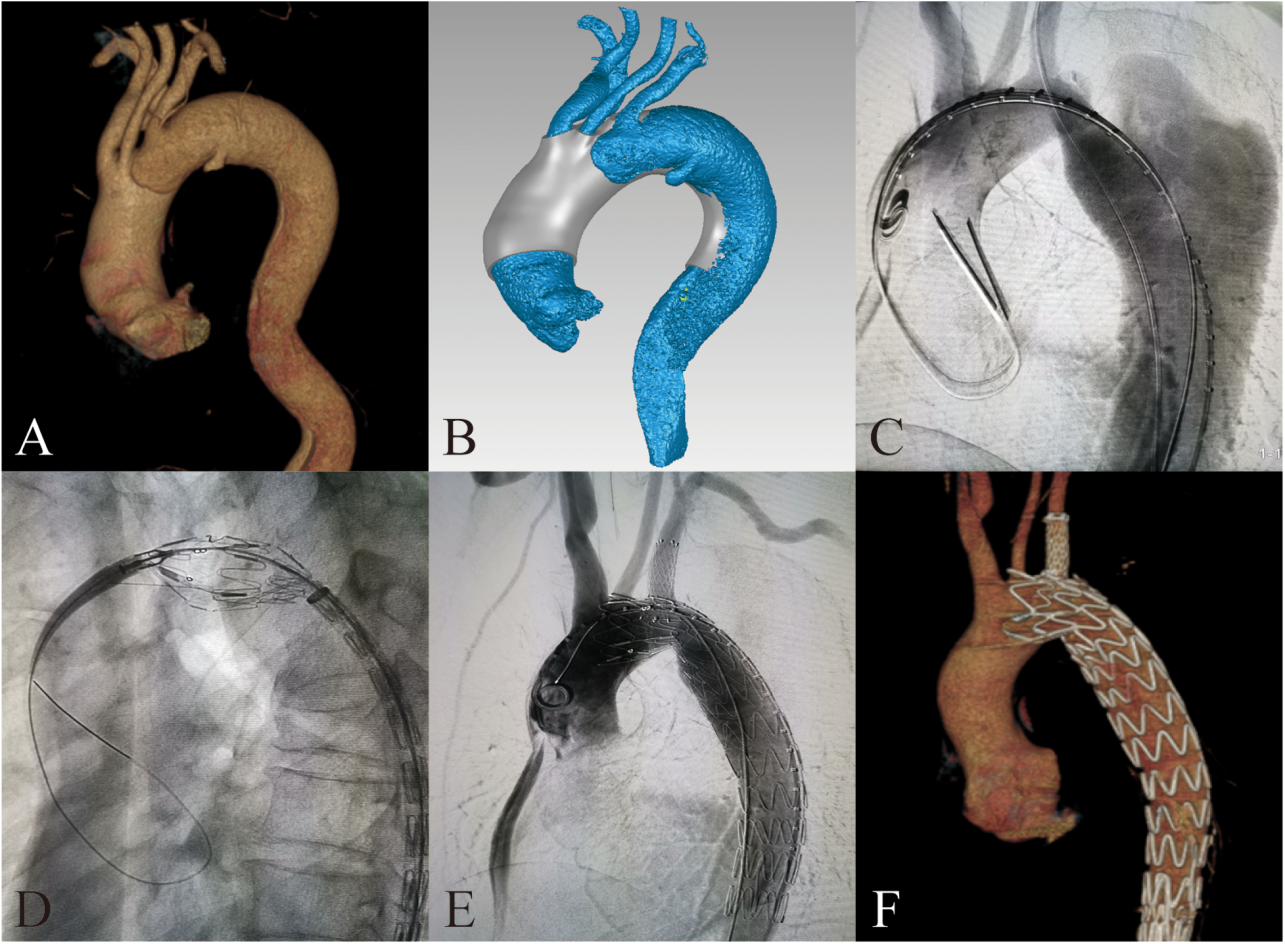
3D-printing assists in fabrication of PMSG with short bridging stents to repair aortic arch dissection. (A) Preoperative 3D reconstruction of the aortic arch dissection based on CTA. (B) Design of 3D-printing model. (C) Preoperative DSA imaging of the aortic arch dissection. (D) Delivery sheath enter into the fenestration. (E) Postoperative DSA imaging of the aortic arch dissection. (F) 3D reconstruction of the aortic arch dissection at 3 months after surgery.
